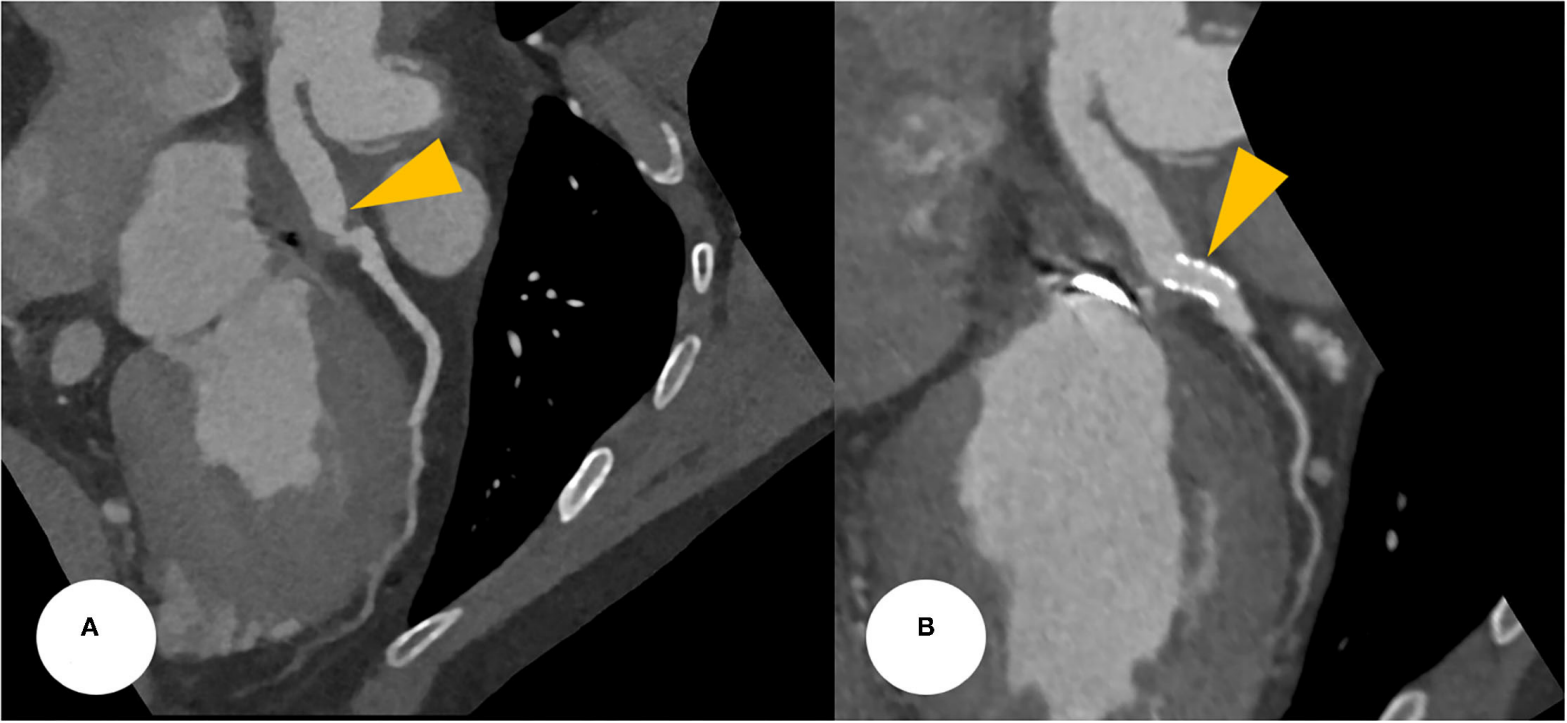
(A) CCTA at the postoperative period found a focally narrowed and kinked lesion at the anastomotic site between the Cabrol-type graft and LMCA (a yellowish arrowhead). (B) After a successful PCI, CCTA was evaluated, revealing a stent deployment state between the conduit and LMCA with good patency (yellowish arrowheads). CCTA, coronary computed tomography angiography; LMCA, left main coronary artery; PCI, percutaneous coronary intervention.
